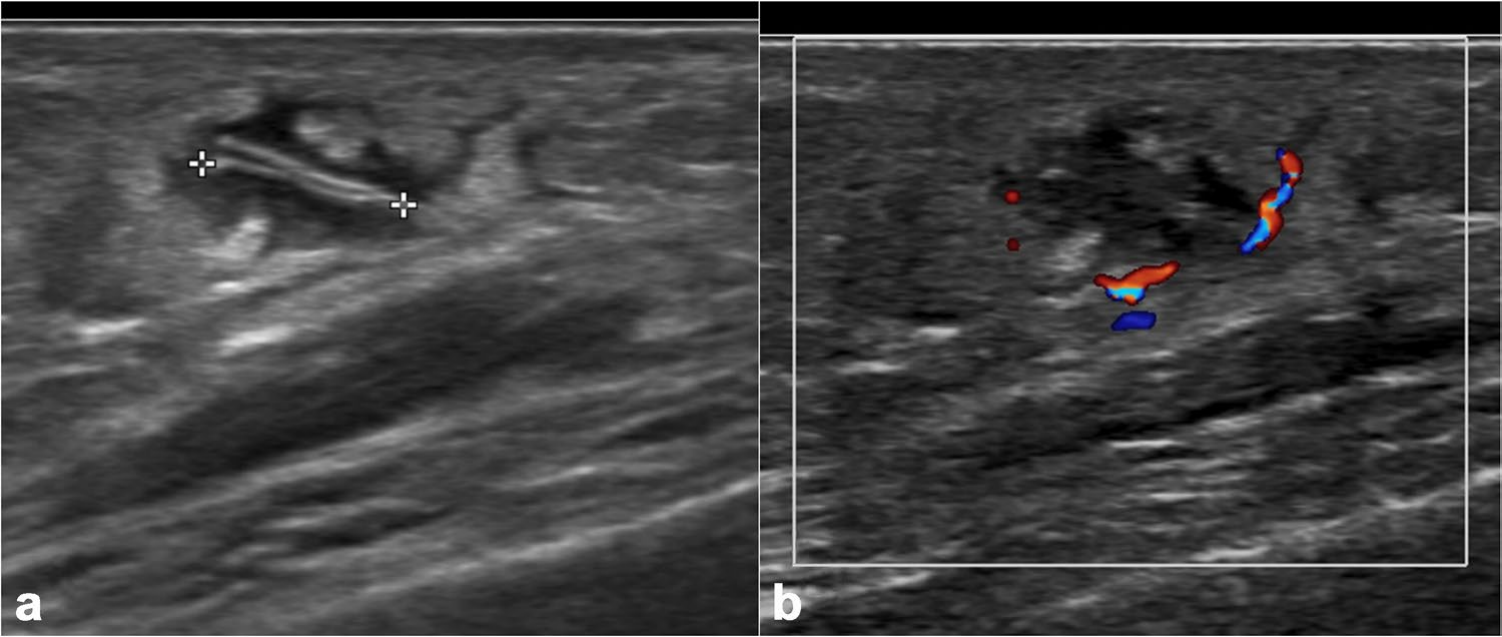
Fourteen-year-old boy with left thigh subcutaneous foreign body granuloma after accident. Grayscale ultrasound (a) demonstrates an 8-mm linear hyperechoic foreign body with surrounding hypoechoic foreign body granuloma and surrounding hyperemia on color Doppler image (b). The patient was asymptomatic and not removed; thus, the exact nature of this foreign body is unknown
